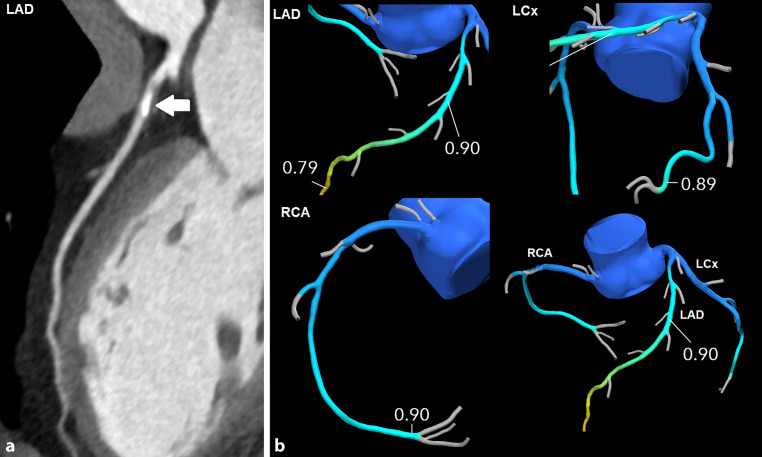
Example of a patient with a an intermediate coronary stenosis in the left anterior descending artery (LAD) with b corresponding CT-FFR analysis negative for ischaemia. No invasive coronary angiography was performed. Please note that the CT-FFR value decreases along all three coronary vessels, despite absence of other focal stenoses
